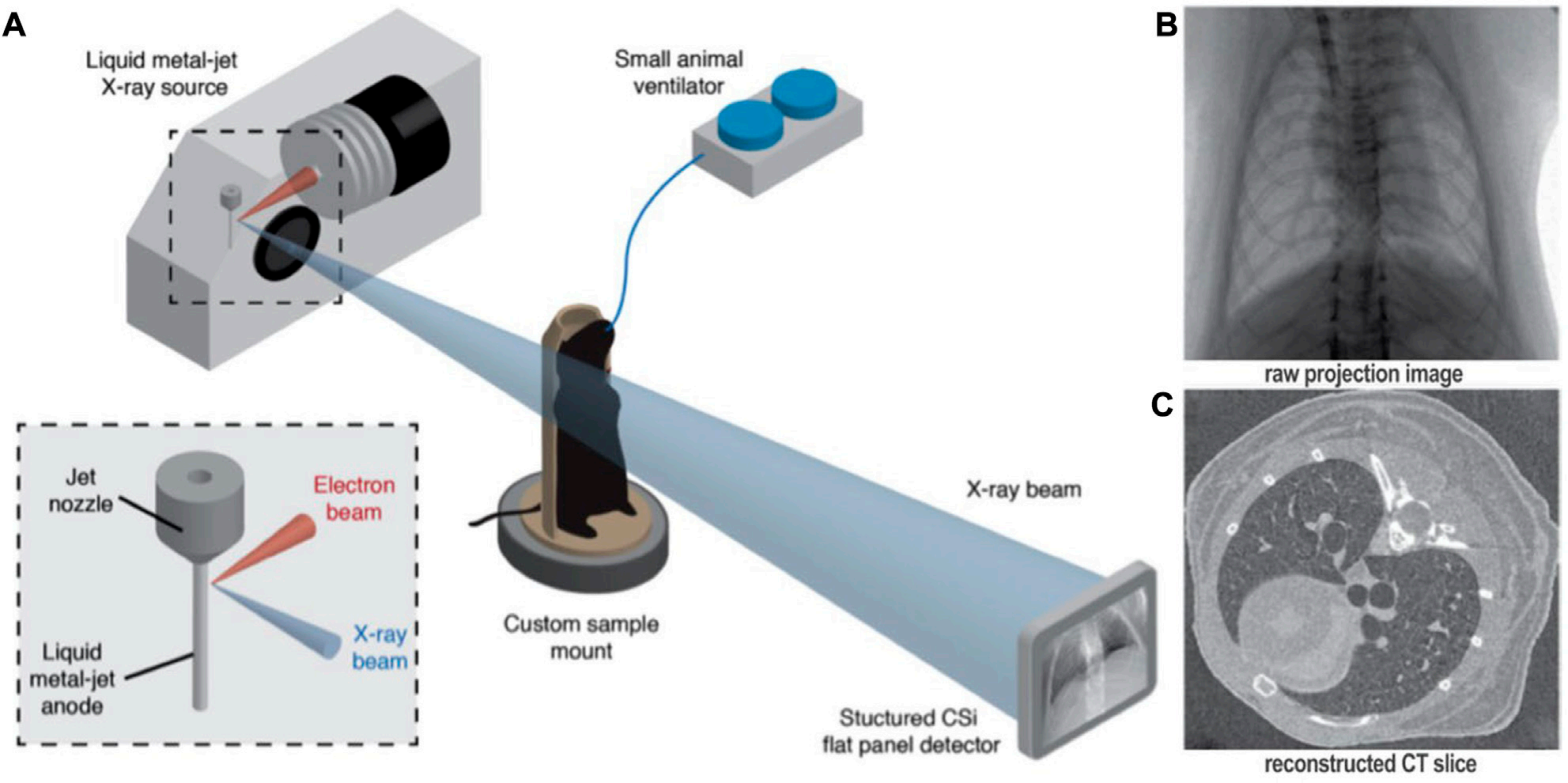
Four-dimensional x-ray velocimetry (4DxV) technique for regional lung function assessments [reprinted from Murrie et al. (2020)]: (A) Experimental image acquisition setup using a liquid-metal-jet microfocus X-ray source, introduced by Murrie et al. (2020) for *in vivo* regional lung function measurements; (B) Raw 2D projections acquired over 360° using the proposed image acquisition setup; (C) Reconstructed CT images from the binned projections to produce 4D CT dataset.
